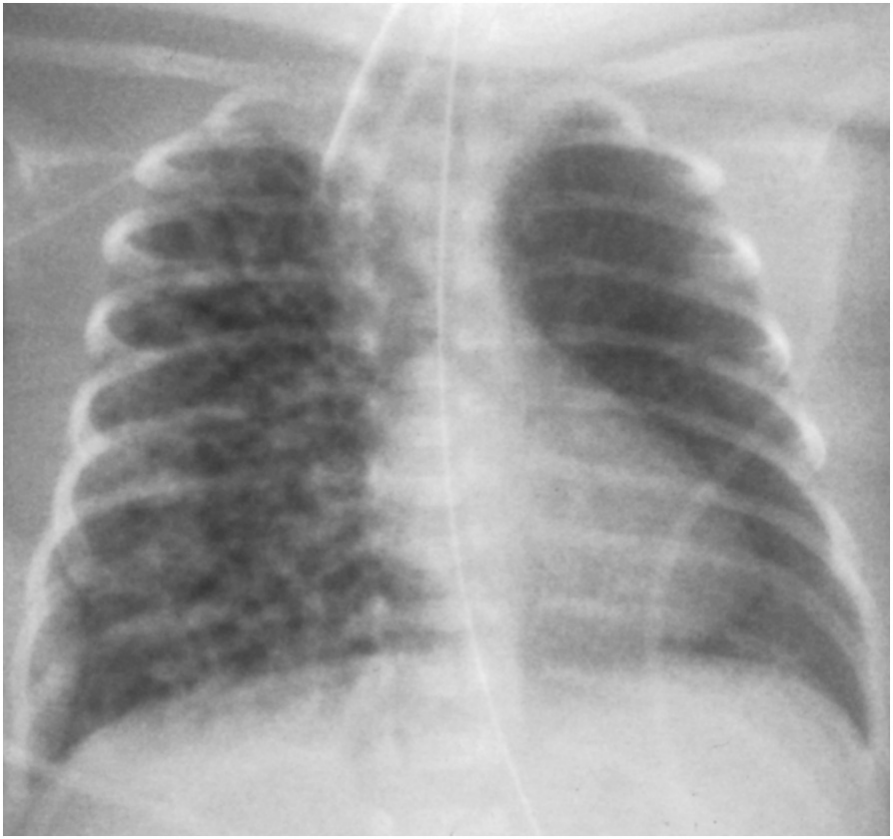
A chest X-ray obtained on postnatal day 6. The chest X-ray shows diffuse pulmonary interstitial emphysema over the entire right lung field.
